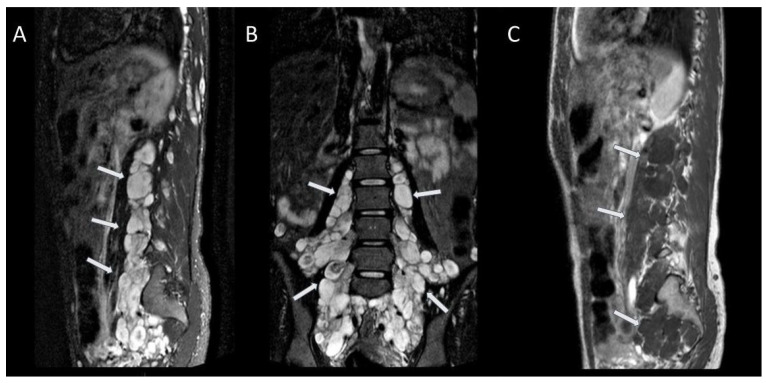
Voluminous plexiform neurofibromas (arrows) in the lumbar, pre-sacral, and pelvic regions. Sagittal STIR (A); coronal STIR (B); sagittal T1-weighted FSE after gadolinium administration (C).
